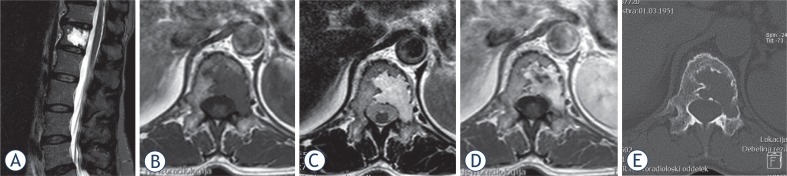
MR and CT images of the patient. The pathologic lesion in 12^th^ thoracic vertebra that fills the majority of the vertebral body is seen as a hyperintense lesion on sagittal fat suppressed (STIR) MR image (A). The lesion appeared homogeneously hypointense on pre-contrast axial *T1 w* SE MR image (B) and homogeneously hyperintense on axial *T2 w* TSE MR image (C). Post-contrast axial *T1 w* SE MR image shows marked enhancement of the lesion that extends to the left pedicle (D). The aggressiveness of the lesion was confirmed with subtle signs of cortical bone disruption seen on CT (E). However, the lesion lacked typical thickened trabeculae what brought a diagnosis of haemangioma into a question.
